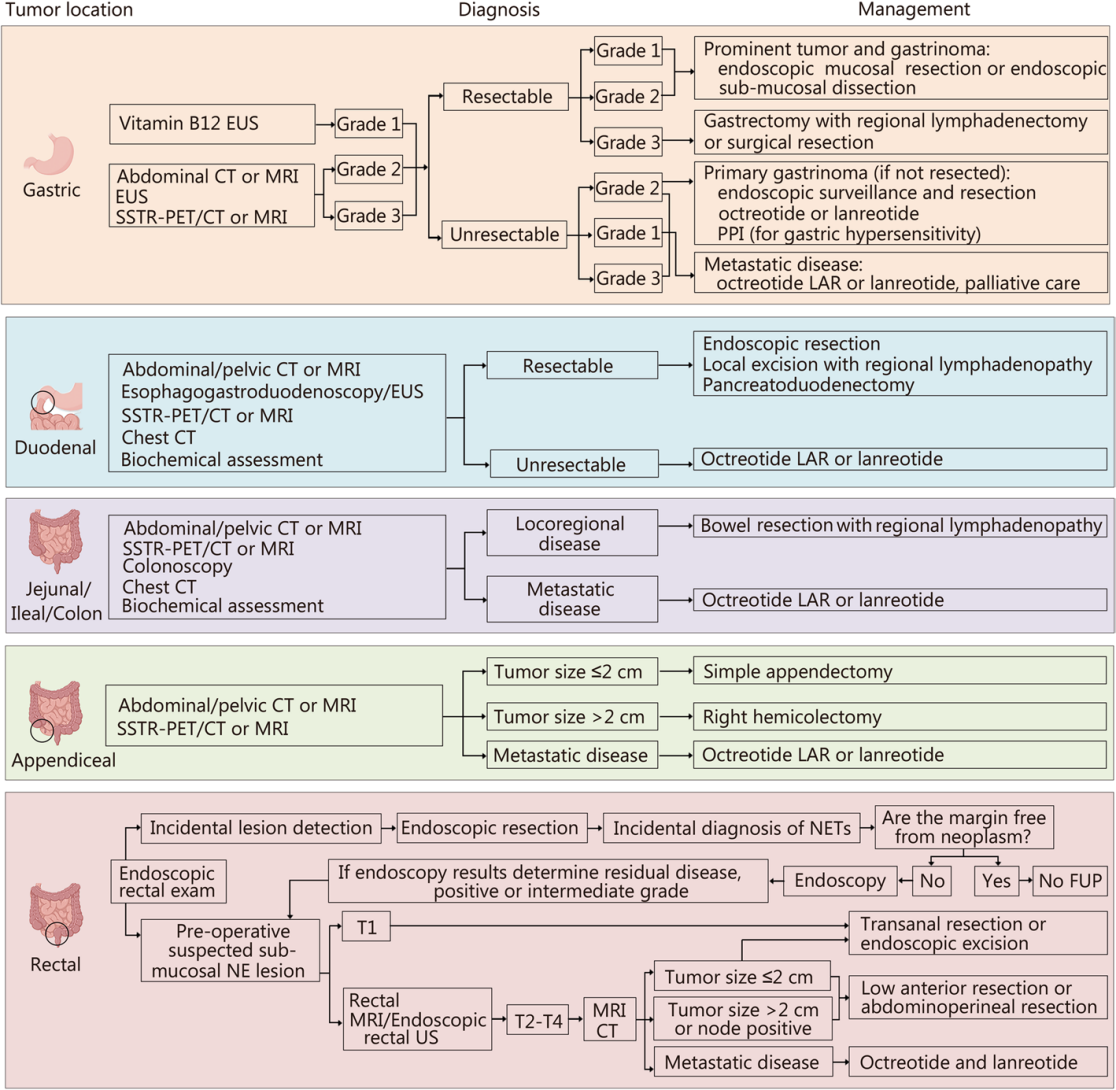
The diagnosis and management of GI-NENs. In different locations of the gastrointestinal tract, including the stomach, colon, and rectum, among others, tumors have been observed. Different strategies can be utilized to diagnose these tumors, including biochemical characteristics, MRI, biopsy, and endoscopy. According to the size of the tumor and grade, surgical resection can be recommended. Hence, the management of these tumors differs based on their origin, tumor size, and tumor grade. EUS endoscopic ultrasound, US ultrasound, CT computed tomography, MRI magnetic resonance imaging, SSTR-PET/CT somatostatin receptor-positron emission tomography/computed tomography, NE neuroendocrine, FUP follow-up, PPI proton pump inhibitor, LAR long-acting release, PRRT peptide receptor radionuclide therapy, T tumor, NETs neuroendocrine tumors, GI-NENs gastrointestinal neuroendocrine neoplasms
